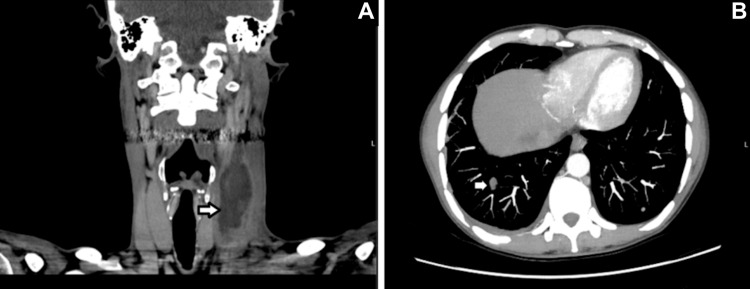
CT neck and thorax before anticoagulation CT neck (A) shows a thrombus within the left internal jugular vein measuring 2.6 x 2.6 x 8.2 cm (white arrow). CT thorax (B) shows a right basal cavitating lesion, which is concerning for septic embolization (white arrow).
